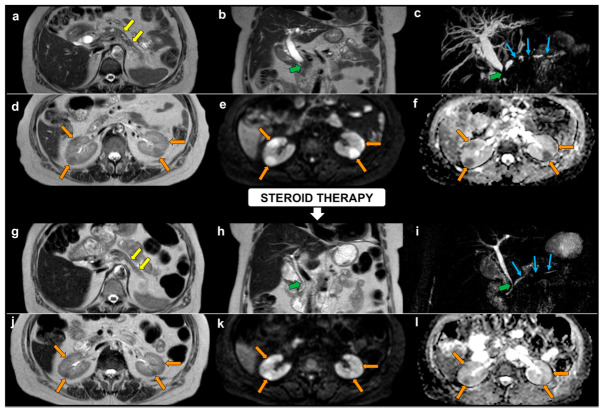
The same patient as in the previous case, a woman with known IgG4-SC and diffuse AIP, who presented one year later with a new episode of painless obstructive jaundice and mild renal function impairment. (a–f) MRI was performed including (a,b,d) T2WI, (c) coronal MRCP with MIP reconstruction, (e) DWI (b = 800 s/mm^2^) and (f) ADC map. A new severe stricture of the distal CBD with circumferential thickening is seen (green arrow), causing marked upstream biliary dilatation, but on this occasion without stenosis of the intrahepatic bile ducts, consistent with cholangiographic type 1 IgG4-SC. An atrophic pancreas is identified (yellow arrows) due to relapsing AIP, with multisegmental MPD strictures and dilatations (blue arrows). Note also the newly developed bilateral renal cortical lesions, hypointense on T2WI and with marked restricted diffusion (orange arrows), which, in the clinical context, were highly suggestive of IgG4-related tubulointerstitial nephritis. (g–l) Follow-up MRI after one month of glucocorticoid therapy shows a dramatic response, with resolution of both the concentric thickening and narrowing of the distal CBD (green arrow) and the upstream biliary dilatation, as well as significant improvement of the multisegmental MPD strictures (blue arrows). A significant decrease in size of the renal lesions with mild residual restricted diffusion is also observed (orange arrows), confirming the suspicion of IgG4-related tubulointerstitial nephritis. Post-treatment pancreatic atrophy is evident as well, more severe than on the previous MRI (yellow arrows).
